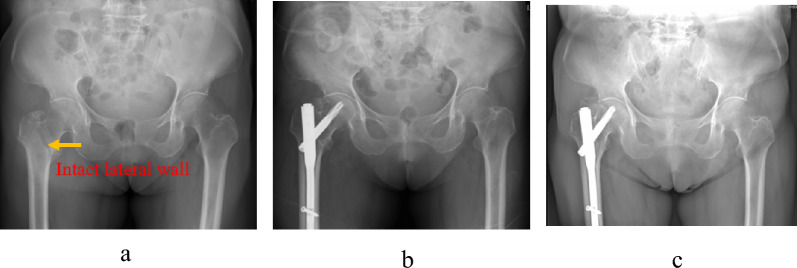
A 67-year-old female was treated for a right intertrochanteric fracture without lateral wall fracture (AO type A1.1) via closed reduction and internal fixation. a Radiograph of right hip showing intertrochanteric fracture without lateral wall fracture. b immediate postoperative radiograph showing sound reduction and after intramedullary nail. c three years follow-up radiograph showing bone union and no varus deformity
